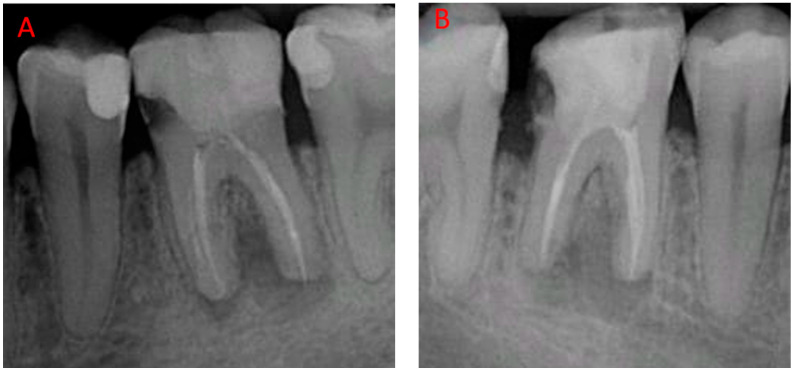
Initial periapical radiographs: (A) 3.6 and (B) 4.6. Both teeth show poor prognosis due to large periapical lesions from endodontic failures.
